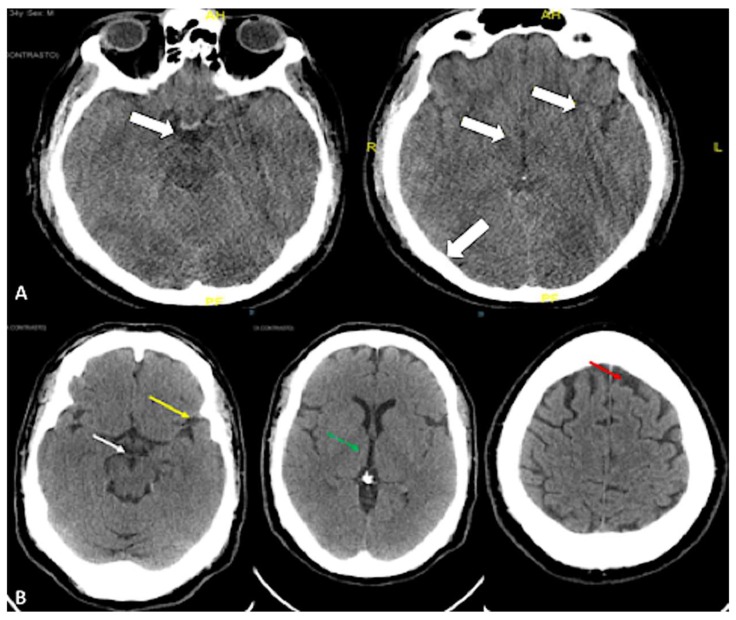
(A) The axial computed tomography (CT) scans evidence diffuse edema with cortical sulci, Sylvian fissure, third ventricle, and perimesencephalic cistern compressed but still visible. White arrow on the left scan shows the perimesencephalic cisterns, while white arrows on the right scan show the cortical sulci, third ventricle, and Sylvian fissure from left to right, respectively. (B) CT scan of a control case. The cortical sulci (red arrow), the perimesencephalic cisterns (white arrow), the Sylvian fissure (yellow arrow), and third ventricle (green arrow with dotted line) are clearly visible. No radiological sign of edema is evidenced.
